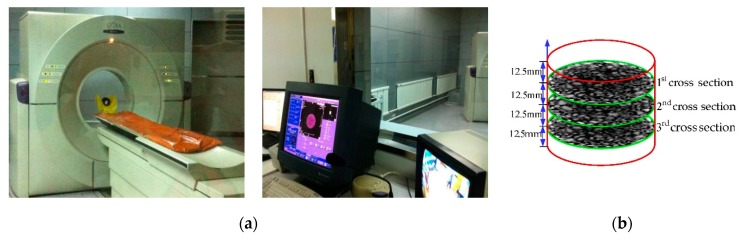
Digital image processing technology. (a) The Philips Brilliance CT scanner, (b) Determination of scanning layers.
